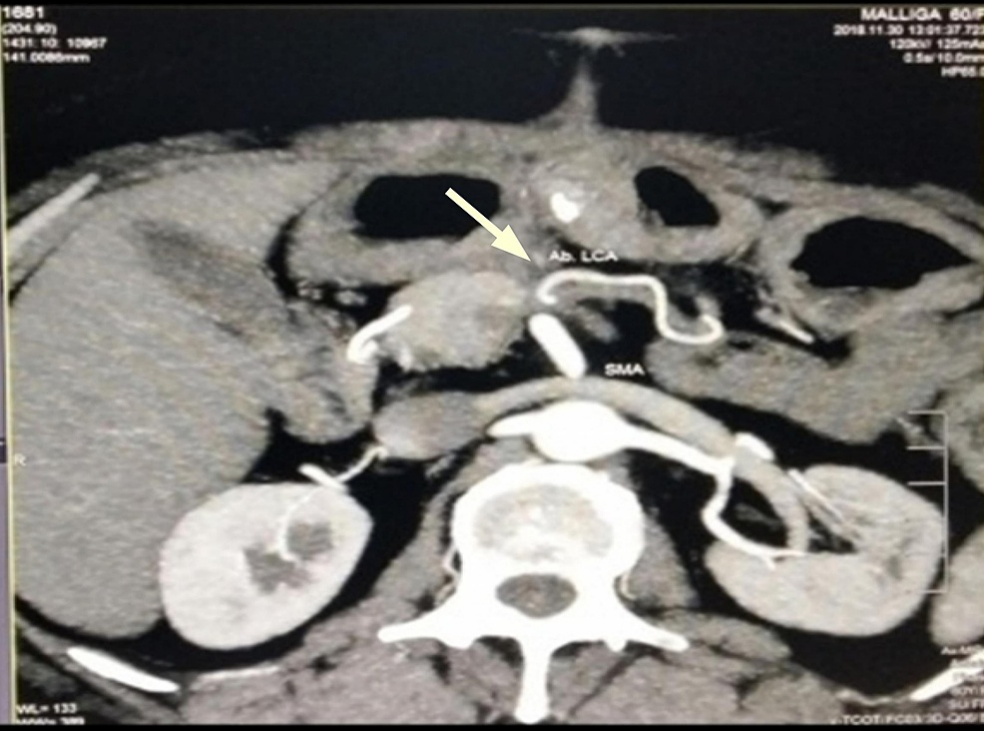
Contrast CT abdomen of ab LCA - coronal section. Contrast CT abdomen of ab LCA with an arrow pointing towards it ab LCA, aberrant left colic artery
